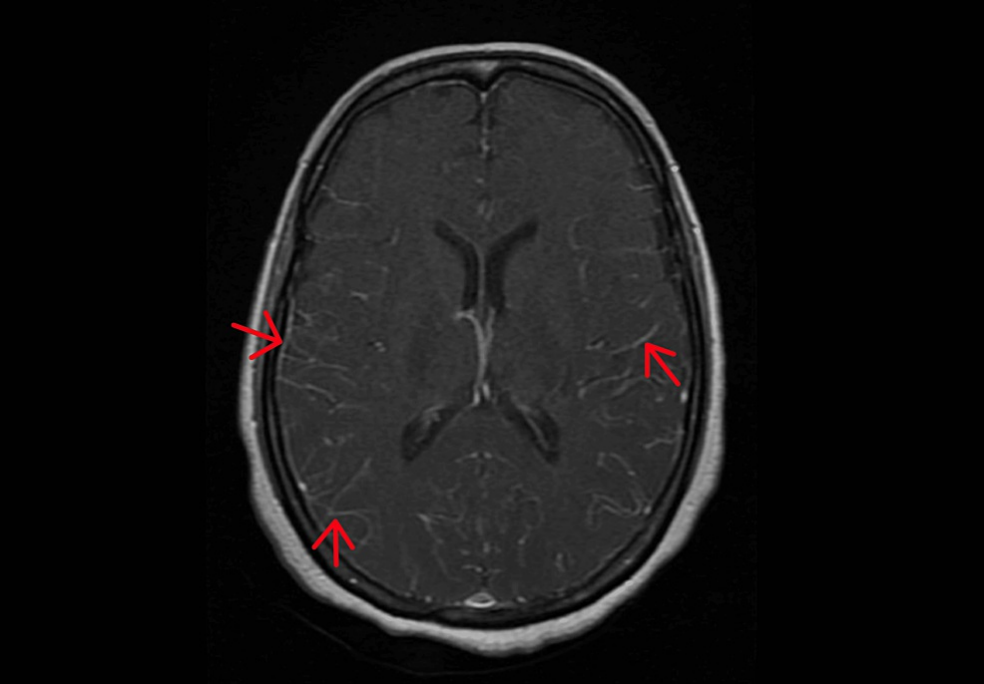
MRI brain showing meningeal enhancement
